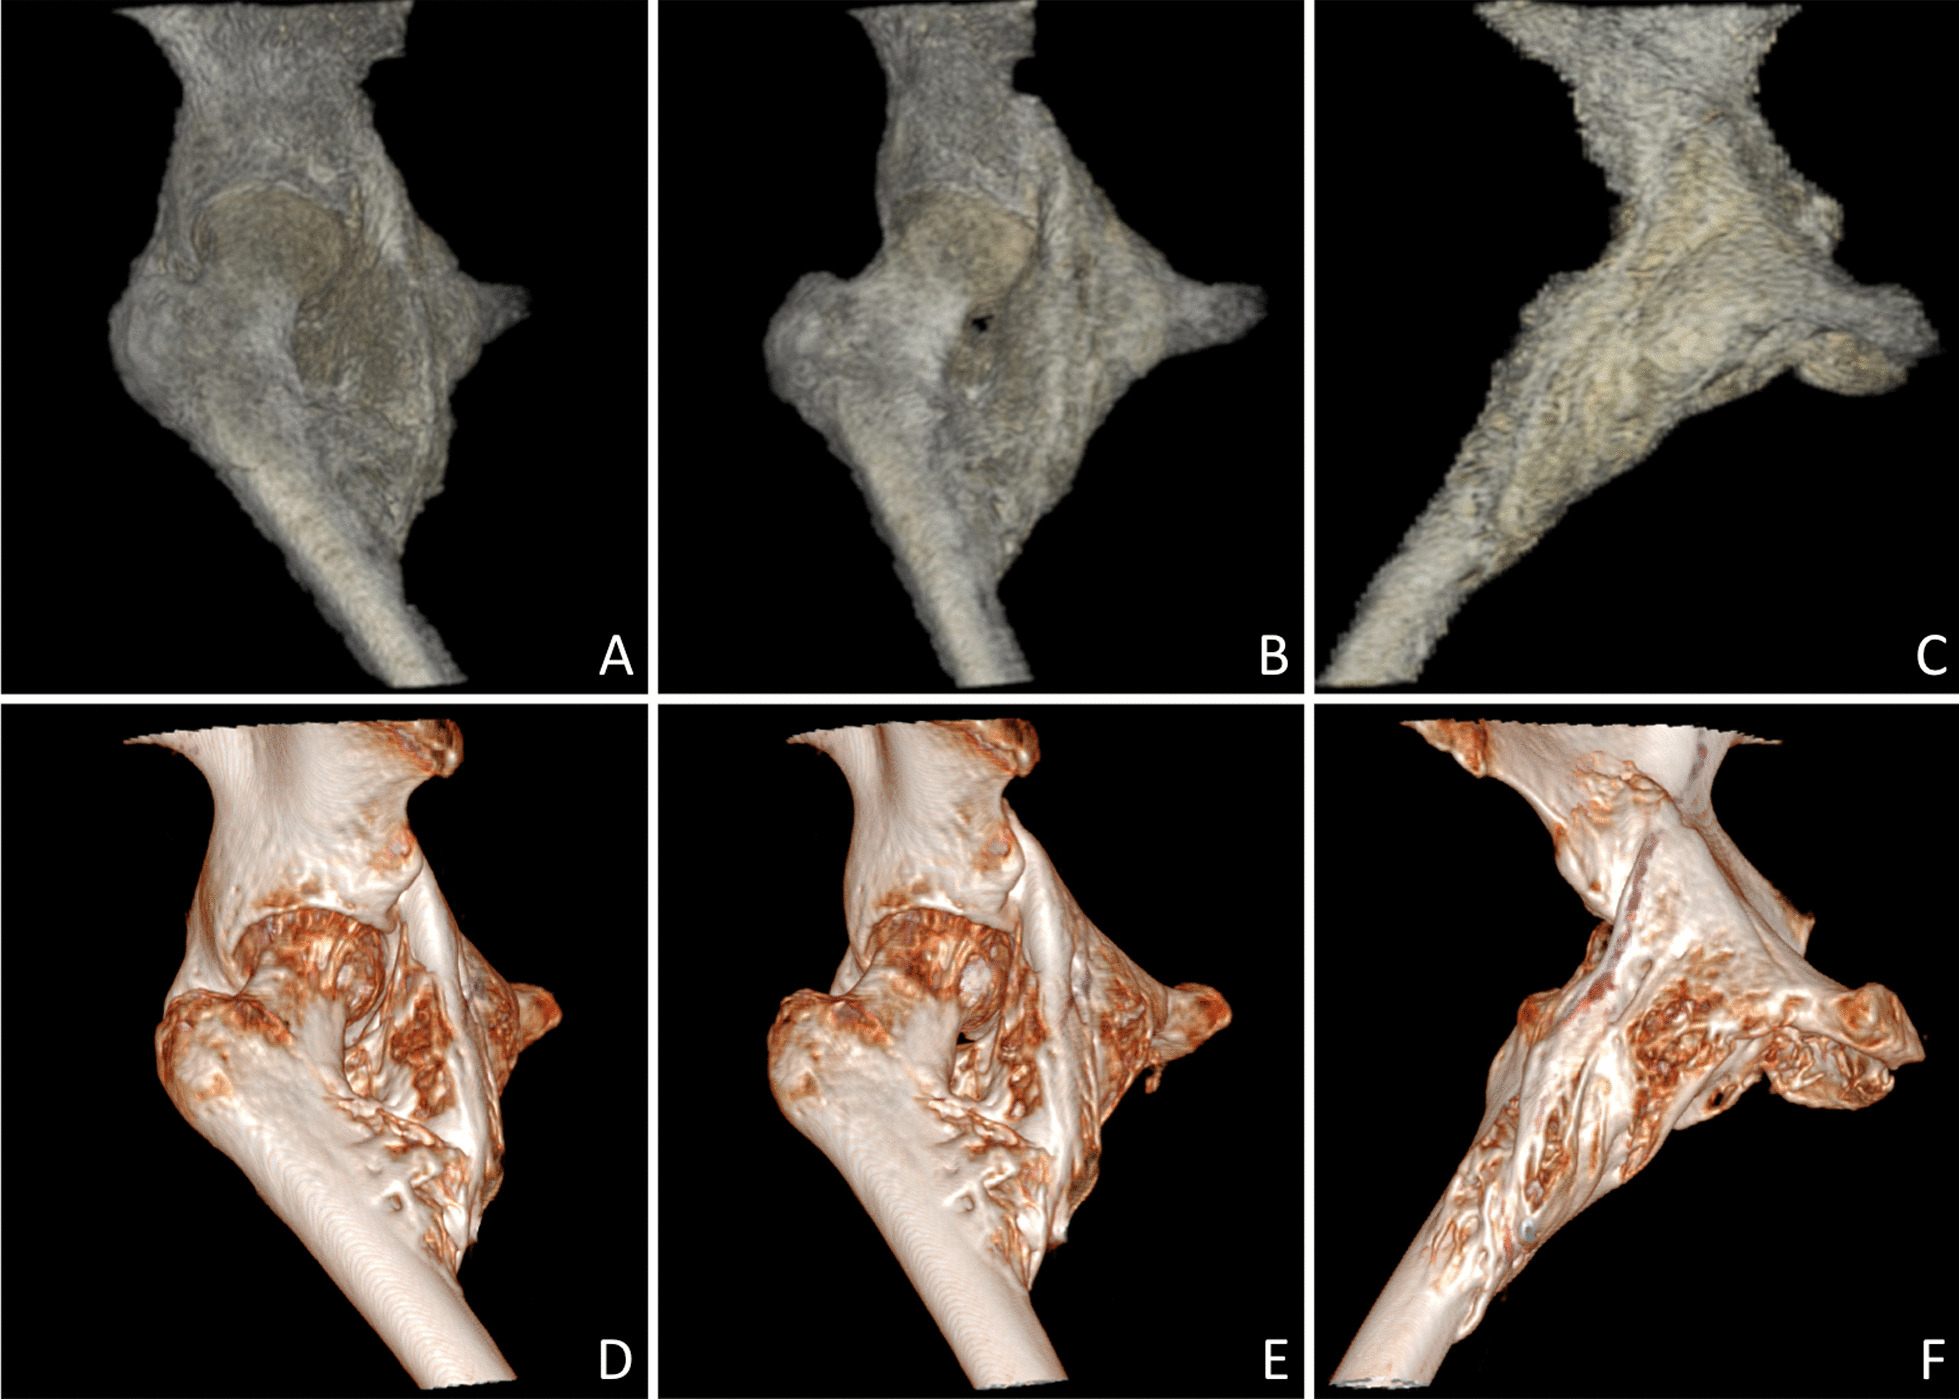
Antero-internal monofragmented NHO of the right hip. 3D volumic reconstructions on ZTE sequence (A–C) and their corresponding images on CT (D–F)
